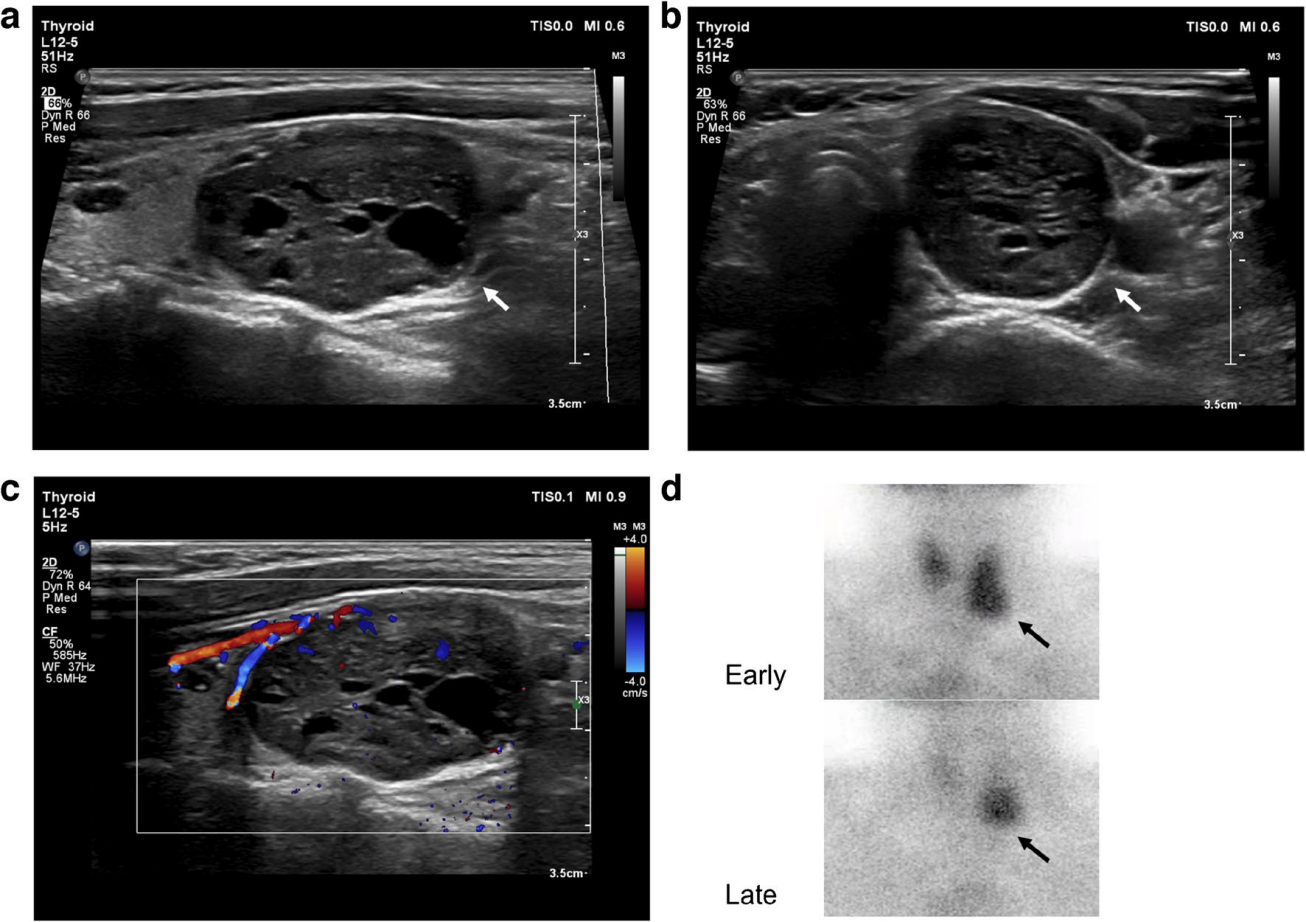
A parathyroid gland adenoma in an 18-year-old girl. a, b Longitudinal (a) and transverse (b) ultrasound images show a nodule (*arrows*) with cystic components below the inferior pole of the left lobe of the thyroid gland. c Longitudinal Doppler image shows a peripheral feeding vessel with an arc of vascularity and internal blood flow. d Technetium-99 m sestamibi scintigraphy shows a focus of intense accumulation at the level of the inferior pole of the left lobe of the thyroid (*arrows*)
